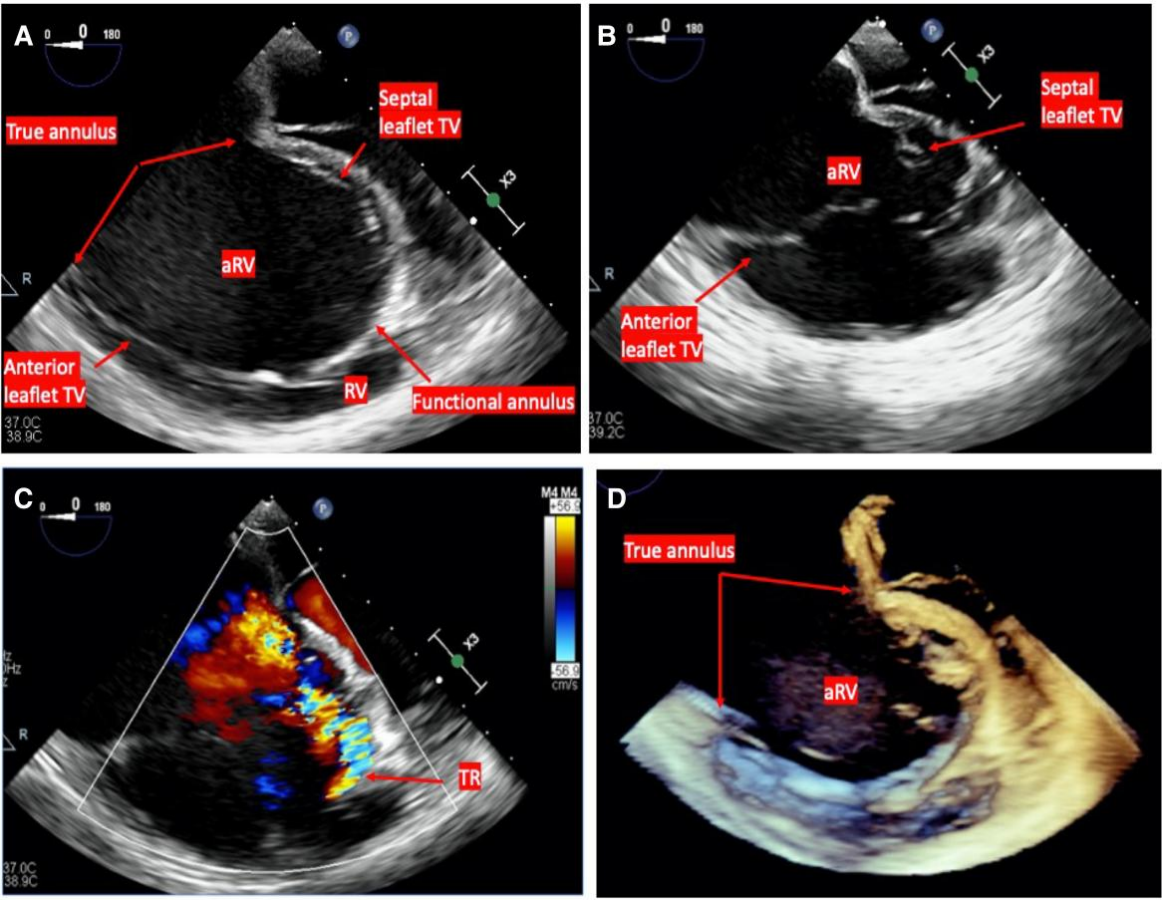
Mid-oesophageal four-chamber view on transoesophageal echocardiography; small right ventricular cavity with leftward septal shift of ventricular septum; the atrialized inlet of the right ventricle is between the true annulus and the functional orifice in diastole (*A*) and systole (*B*). Severe tricuspid regurgitation through the functional orifice (*C*). 3D imaging with dilated atrialized inlet of the right ventricle (*D*). RV, right ventricle; aRV, atrialized inlet of RV; TV, tricuspid valve; TR, tricuspid regurgitation.
